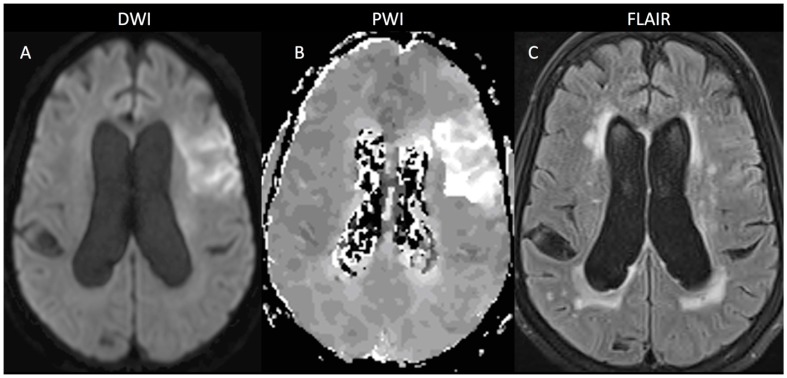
The MRI scan from the time of presentation (pre-treatment) is shown. Panel A is the diffusion weighted image (DWI) demonstrating an acute stroke in the left frontal lobe (radiologic convention). Panel B is a perfusion weighted image (PWI) showing the time-to-peak (TTP) map which demonstrates decreased blood flow in the region of acute ischemia. Panel C shows the FLAIR image which demonstrates that although the acute stroke has not yet developed increased T2 signal intensity, there are many areas of chronic white matter hyperintensity bilaterally.
